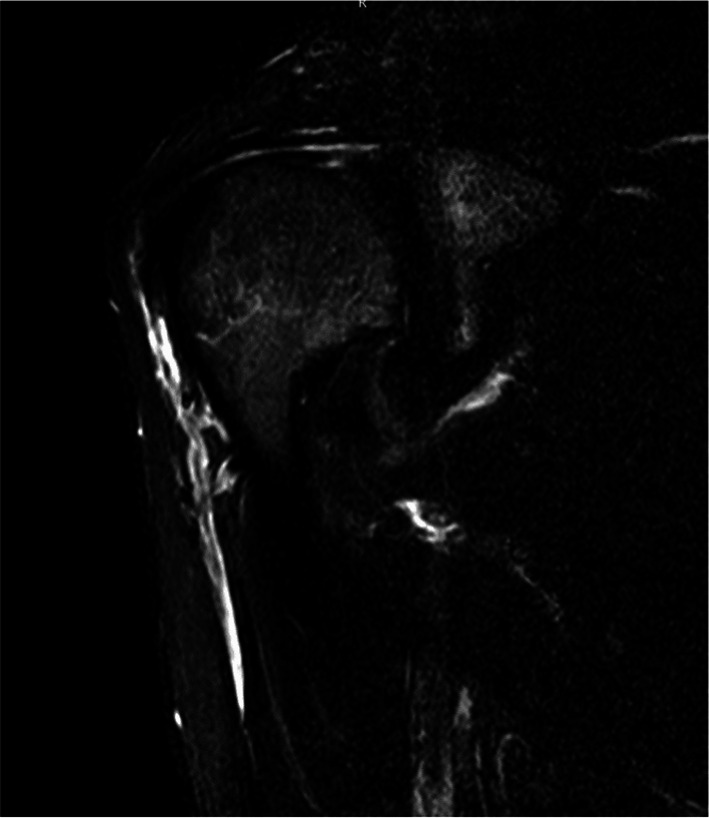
Anterior deltoid atrophy shown by magnetic resonance imaging
